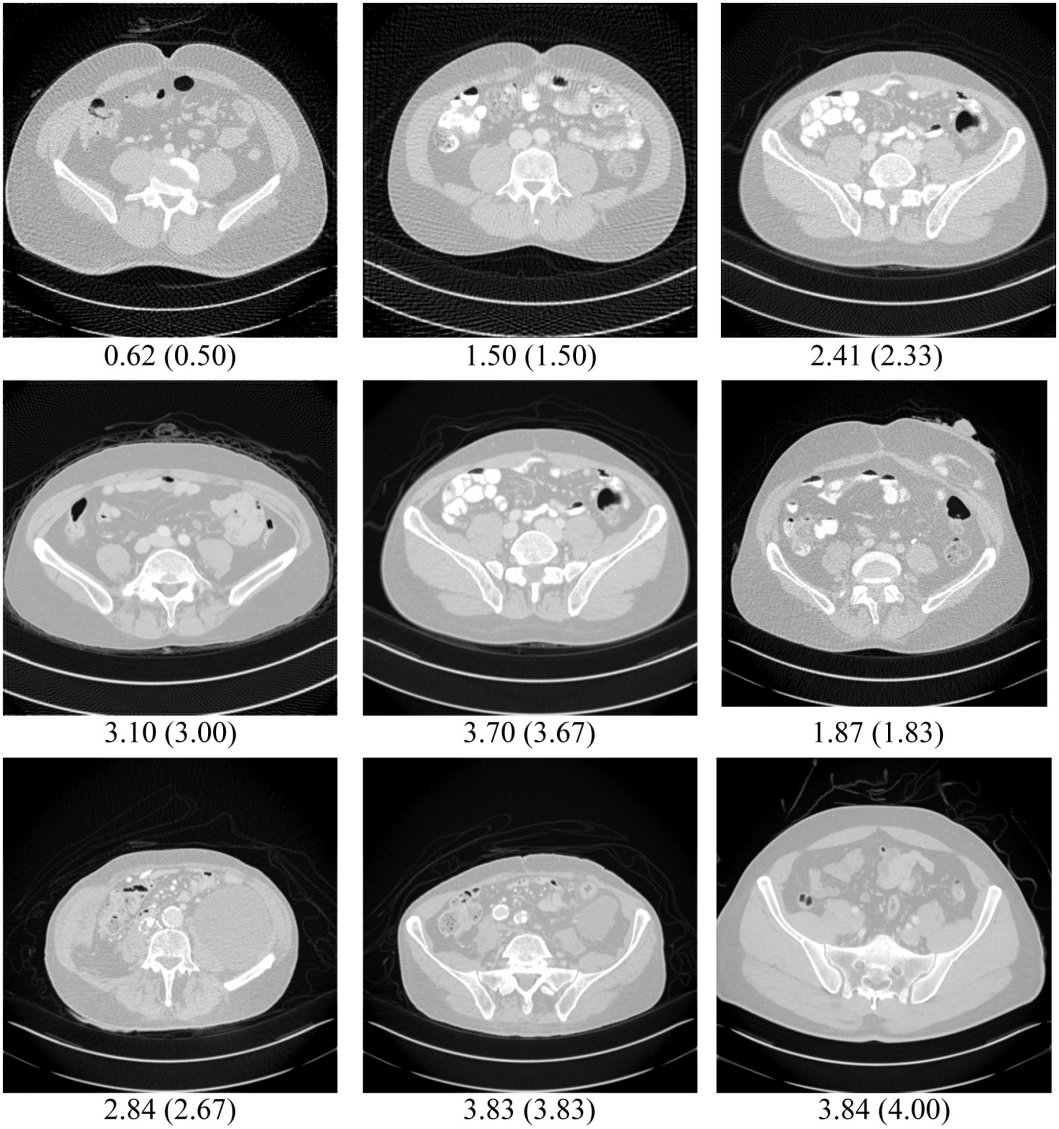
Effectiveness of our TFKT V2 model in accurately assessing the quality of abdominal CT images across diverse IQA scores. Model predictions are in good agreement with the (actual) scores (window width: 1300; level: −800).
